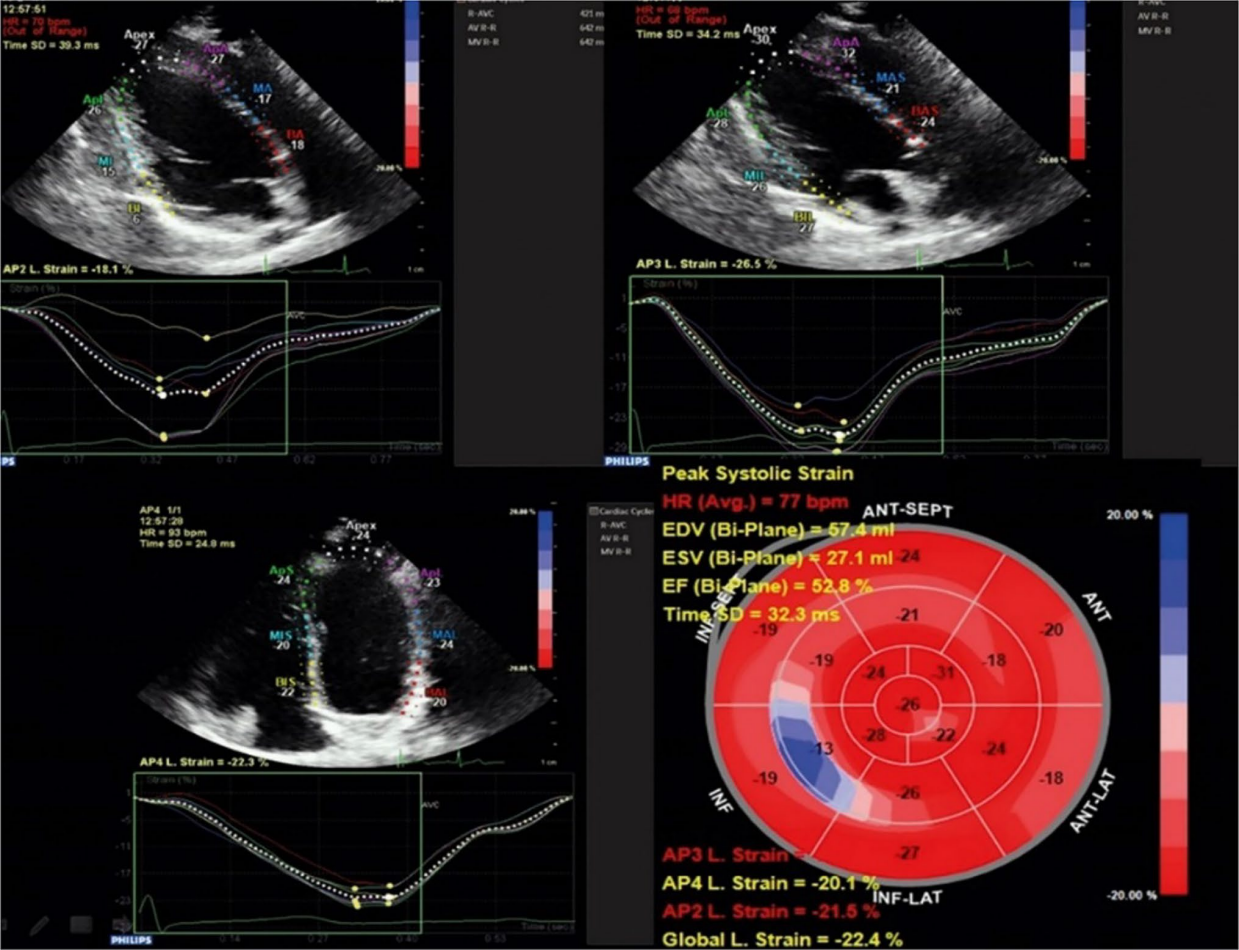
Speckle tracking strain echocardiography segmental analysis of cardiac function
